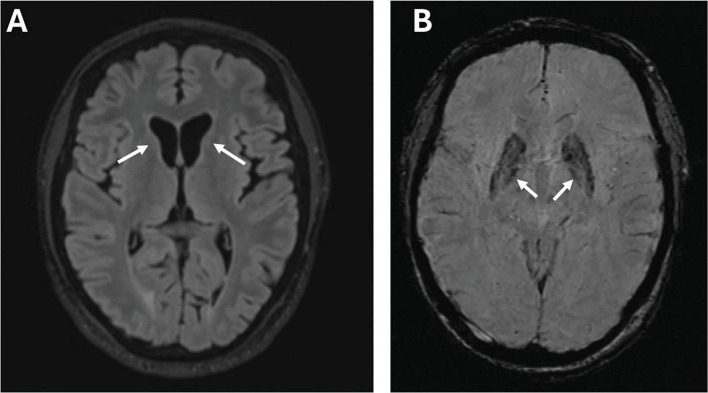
Imaging features of Case D. Brain MRI of case D at age 53 years, showing marked atrophy of the caudate nuclei bilaterally in a T1-weighted scan (arrows) (A); susceptibility-weighted images (SWI) sequence showing abnormally increased iron deposition in the globus pallidus (arrows) (B).
